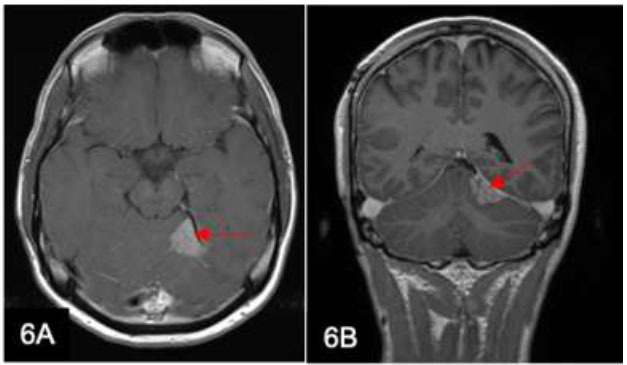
A 28-year-old man diagnosed with pineoblastoma underwent radiotherapy. Axial T1-weighted (w) post-contrast (A) and coronal T1w post-contrast (B) images show an enhancing dural-based lesion arising from the inferior surface of the left tentorium with adjacent leptomeningeal involvement (arrows). This is consistent with leptomeningeal metastasis.
